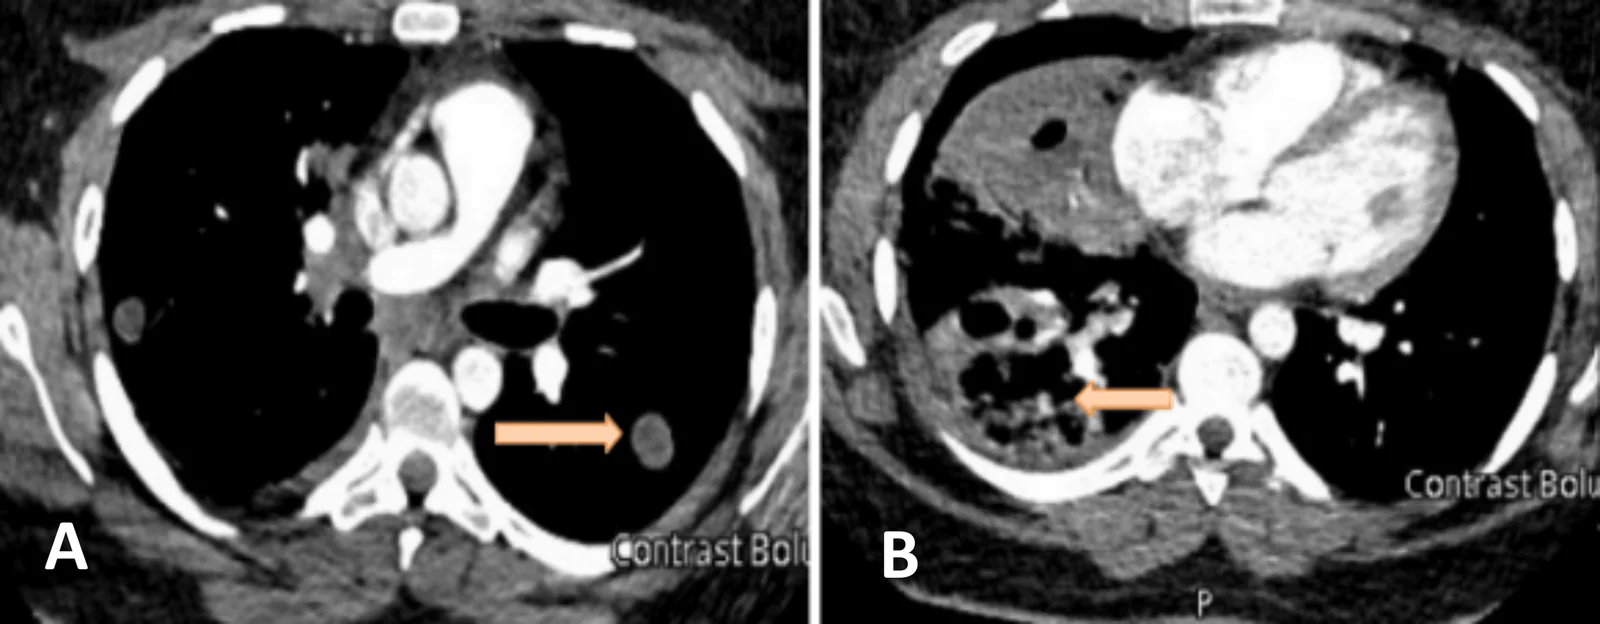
CT scan of the chest on admission: (A) cross-section of the upper lung field and (B) cross-section of the mid-lung field (A) Nodular opacity in the upper left lung field representing an *Echinococcus *cyst. (B) Consolidation with necrosis and cavitary liquefactive changes in the right mid-lung field.
